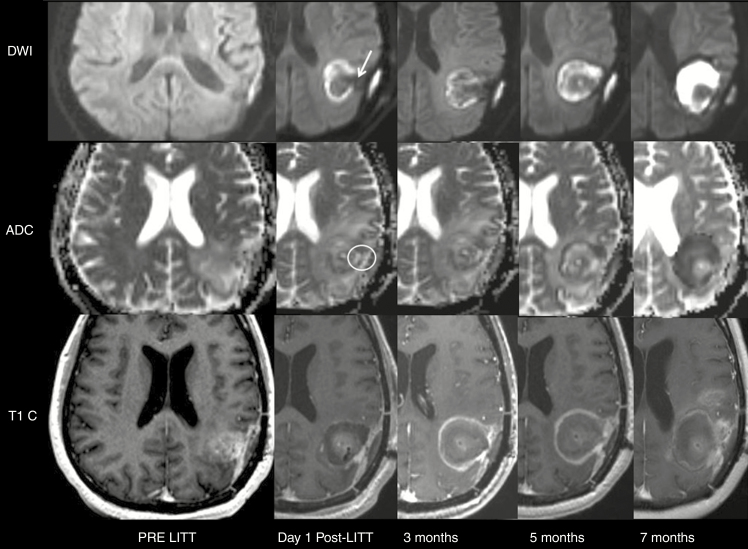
Typical MRI scans before and after LITT in 43-year-old male with GBM with recurrence after LITT: On day 1 post-LITT, there is a discontinuity in the peripheral restricted diffusion (white arrow) with corresponding decreased DWI signal and increased ADC (white circle) within the expected periphery of restricted diffusion 24 h post-LITT. This corresponds to an area of new peripheral enhancement on T1 postcontrast images obtained after 5 months, consistent with recurrence. The site of discontinuity on DWI obtained at day one after LITT predicts the location of recurrence in the subsequent scans in this case.
